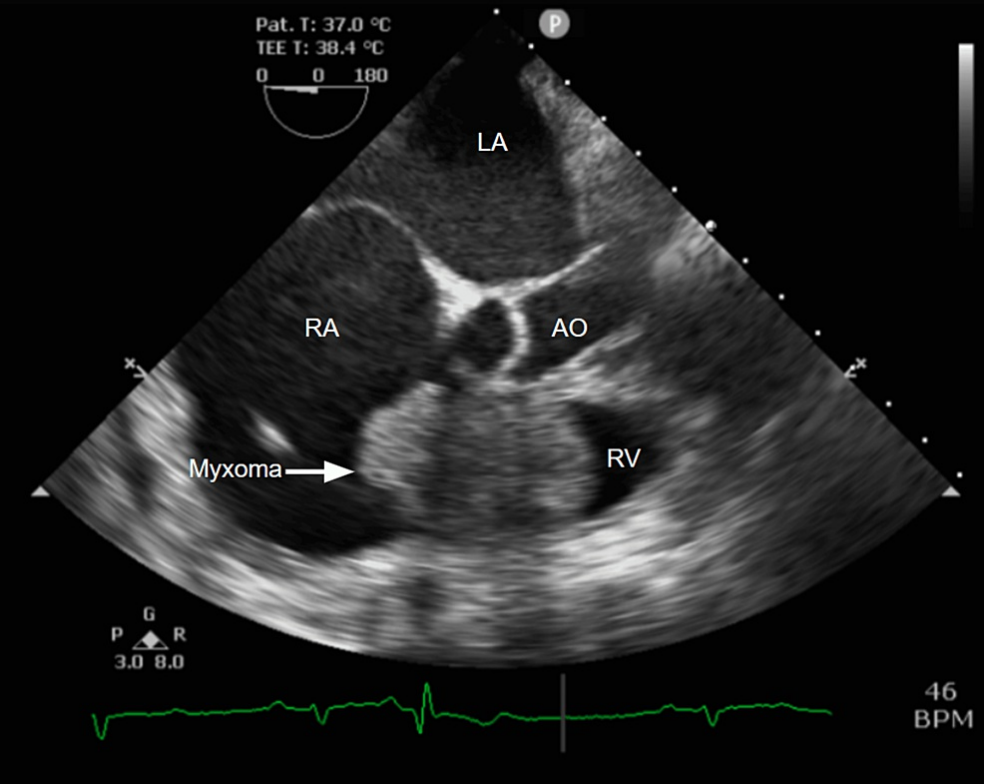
Transesophageal echocardiogram confirms the presence of a large, oval, mobile, and heterogeneous right atrial mass with a smooth surface obstructing the right ventricular inflow AO = Aorta. RA = Right Atrium. LA = Left Atrium. RV = Right Ventricle.
